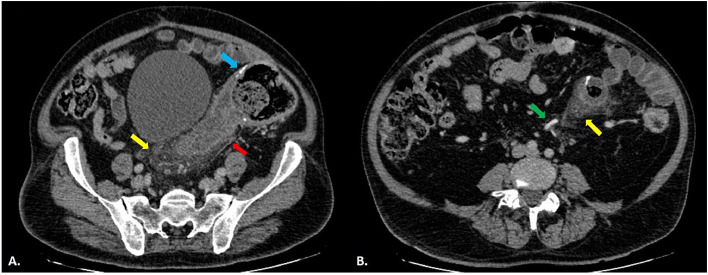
Coronal view of abdominal CT scan, showing long-segment circumferential wall thickening (red arrow).
